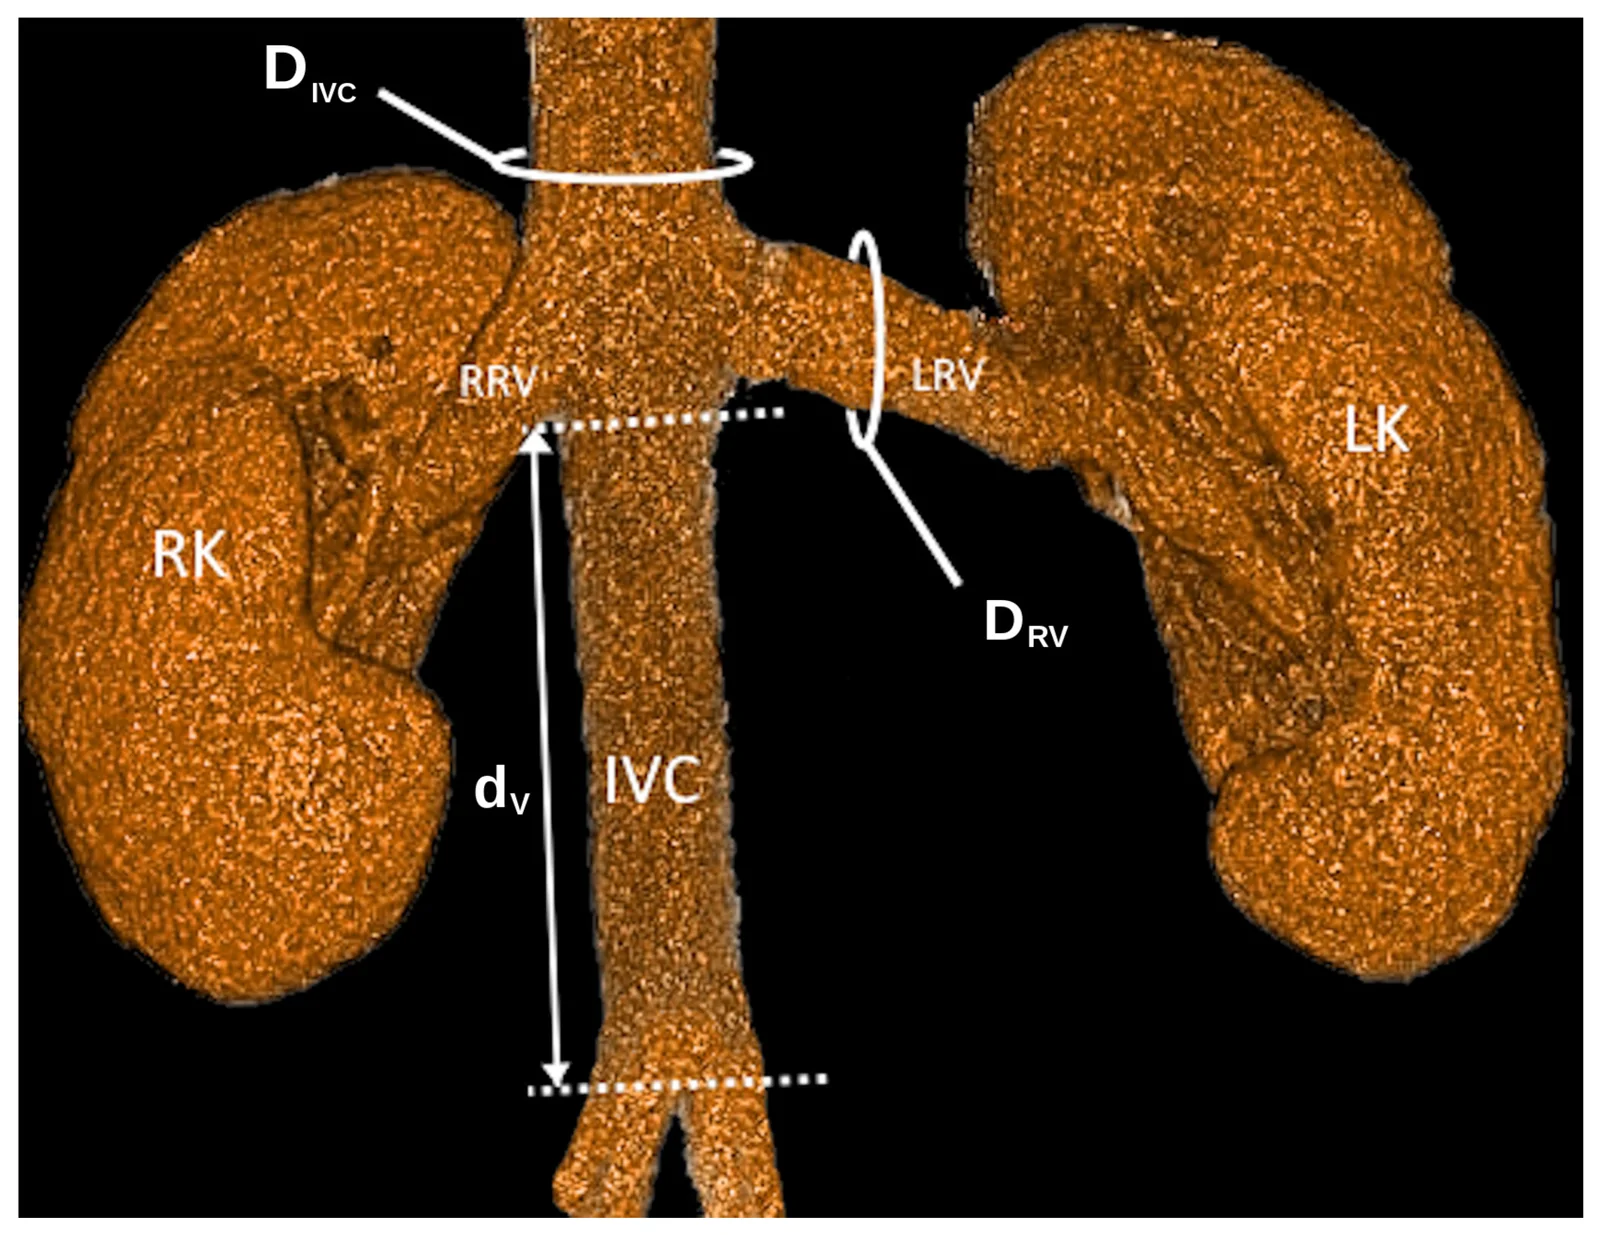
Scheme of the morphometric and topographical measurements, venous side (three-dimensional reconstruction of contrast-enhanced computed tomography, venous phase). Diameter of the inferior vena cava (D_IVC_), diameter of the renal vein (D_RV_) and craniocaudal distance of the termination from the confluence of the common iliac veins (d_V_). IVC, inferior vena cava; RK/LK, right/left kidney; RRV/LRV, right/left renal vein.
